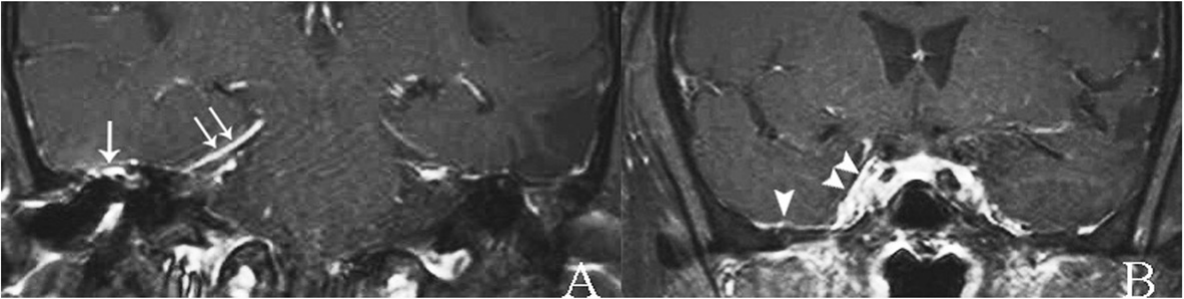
Coronal postgadolinium-pentetic acid spin echo T1-weighted with fat suppression magnetic resonance imaging. A. Abnormal prominent enhanced roof of the middle ear cavity (arrow) and thickened enhanced anteromedial part of right tentorial cerebelli (arrows). B. Abnormal prominent enhanced floor of right middle cranial fossa (arrowhead) and right cavernous sinus (arrowheads).
